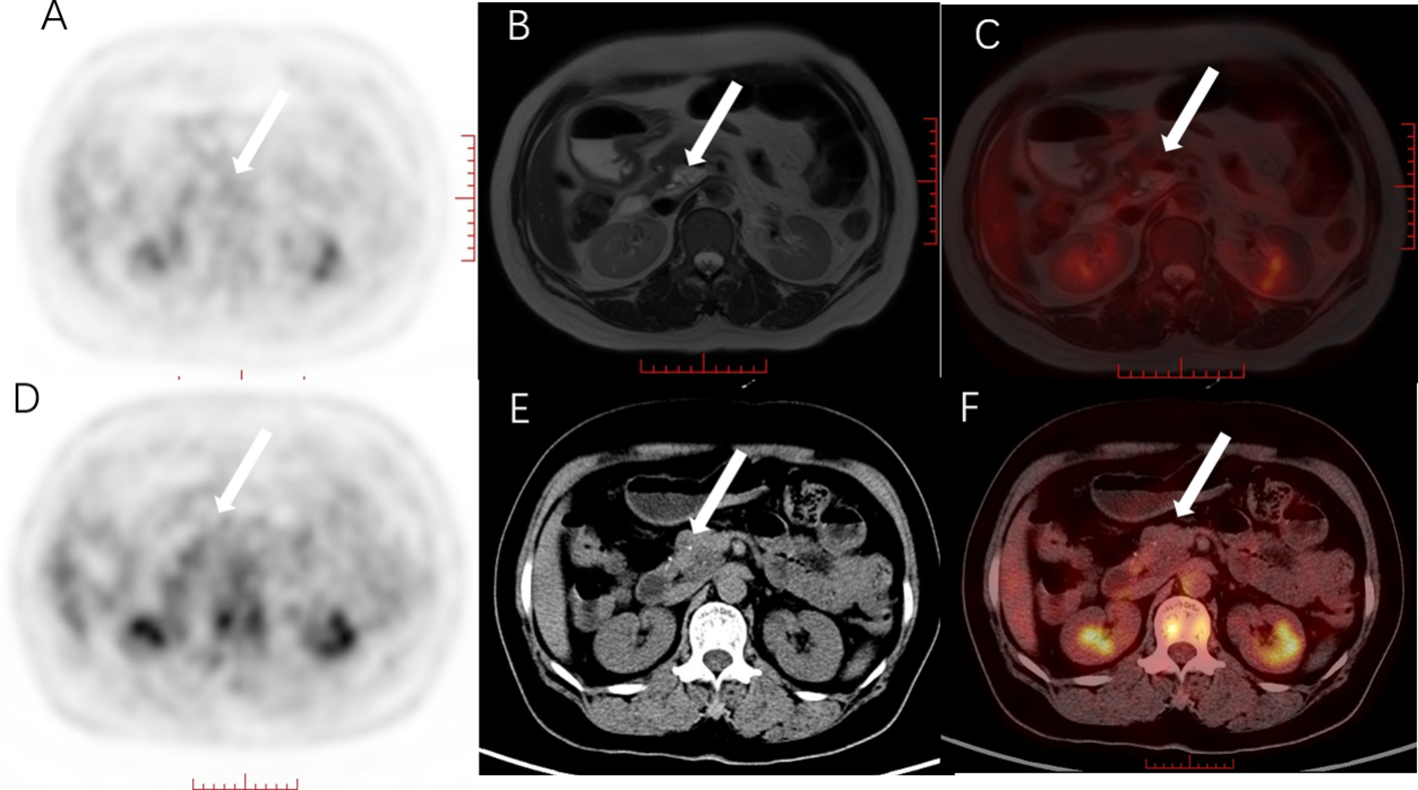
Female, 56 years old, with mucinous cystic tumor of the pancreatic head. (A) PET MIP image, no obvious hypermetabolism was found in the pancreas; (B) MRI T2WI, T2WI showed heterogeneous and slightly hyperintensive cystic-solid lesions; (C) PET/MRI fusion image, there was no metabolism on the fusion image, but A clear display of the cystic and solid components of the lesion is helpful for accurate diagnosis. (D) PET MIP image, suspected hypermetabolic foci in the pancreatic head area; (E) plain CT scan, the pancreatic head lesion was unevenly low-density, and dot-like high-density can be seen in it; (F) PET/CT fusion image, PET/CT fusion images showed no obvious metabolism compared with the pancreatic background. The white arrow shows the location of the lesion. PET/MRI fusion images clearly show the cystic and solid components of the lesions, which is helpful for accurate diagnosis.
